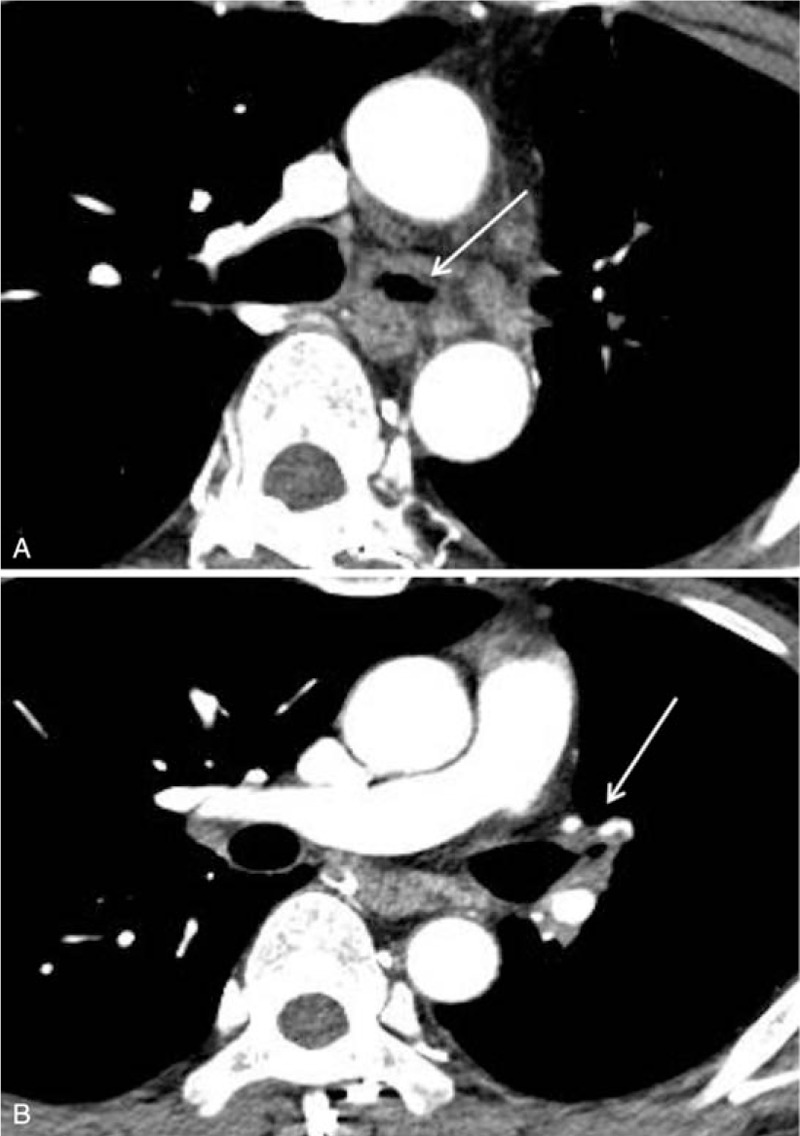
Chest CT findings of late airway complication 4 months post acid ingestion. A, B Luminal narrowing of the left main and left upper lobar bronchi with bronchial wall thickening is seen on chest CT (arrows).
